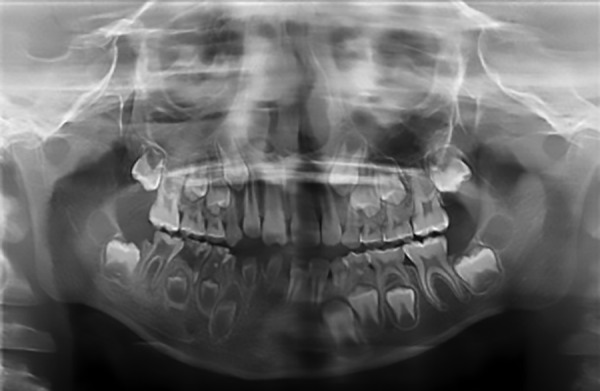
Panoramic radiograph in which deciduous and permanent teeth in the right hemimandibular show large pulp chambers. Agenesis of teeth 41 and 42 is observed, as well as images suggestive of caries on teeth 84, 85, and 46
